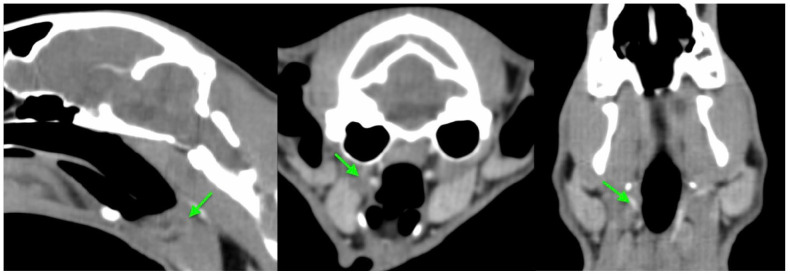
Contrast-enhanced CT multiplanar reconstruction using soft tissue windowing demonstrating lack of tumour reoccurrence 7 months following the surgery. Green arrows show previous location of the mass
